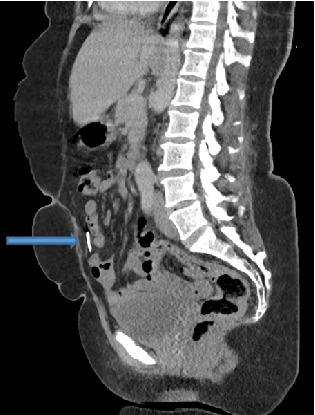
CT scan in sagittal view revealed a surgical blade in the abdomen.
